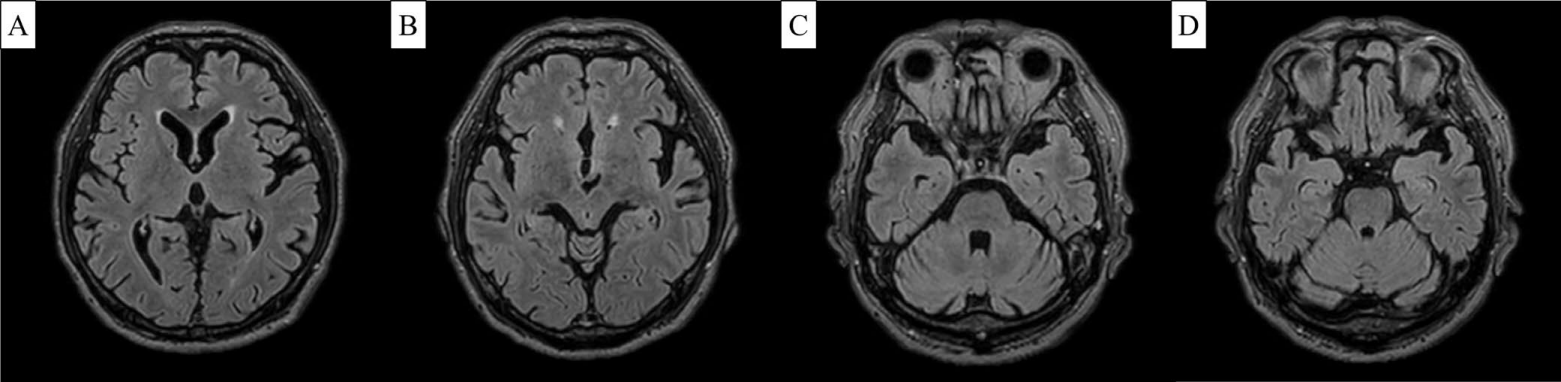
Head magnetic resonance image 16 months after onset (horizontal section, FLAIR images). FLAIR images show that the high signal regions in the bilateral basal ganglia (A), external capsule, thalamus (B), and pons (C, D) have disappeared.
